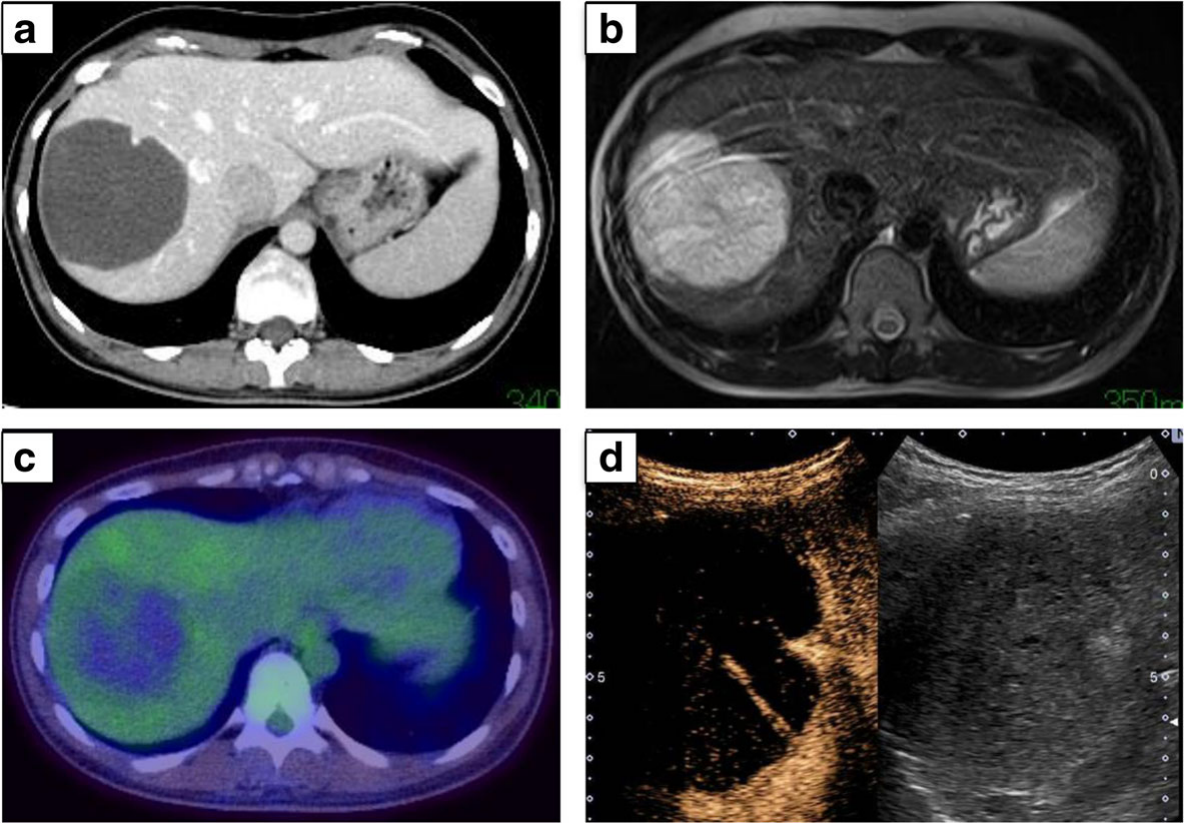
a CT scan showing a lobular, cystic tumor with highly viscous liquid. b MRI showing hyper-intense regions on T2. c FDG-PET showing the lack of uptake in the tumor. d Sonazoid-enhanced ultrasound showing a solid, hypovascular tumor with an enhanced internal partition wall
